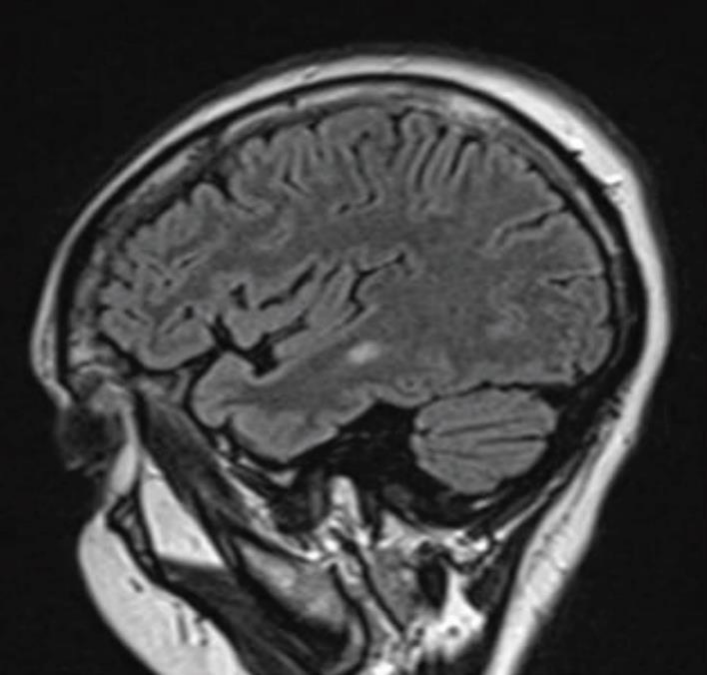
Sagittal T2 FLAIR MRI, series 9, image 5/48. Periventricular left temporal horn lesion.
